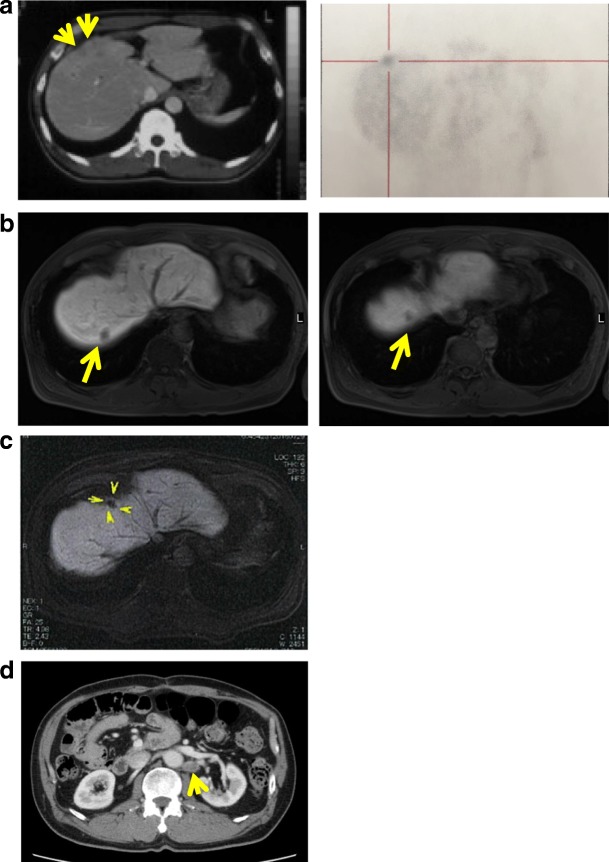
Image diagnosis. a Left: positron emission tomography-computed tomography (PET-CT) findings at 3 months after surgery shows poor contrast enhancement area in S5 (arrows). Right: abnormal ^18^F-fluorodeoxyglucose (FDG) uptake was detected in the same lesion. b Magnetic resonance imaging (MRI) with gadolinium ethoxybenzyl diethylenetriamine penta-acetic acid (Gd-EOB-DTPA) before first microwave coagulo-necrotic therapy (MCN) shows hypointense lesions (arrows) at S7 and S8 in the hepatobiliary phase. c MRI with Gd-EOB-DTPA before second MCN shows hypointense lesions at S4 (arrows) in the hepatobiliary phase. d Current computed tomography image shows undeniable metastasis (arrow) with contrast effect in para-aortic lymph node, but no ascites
